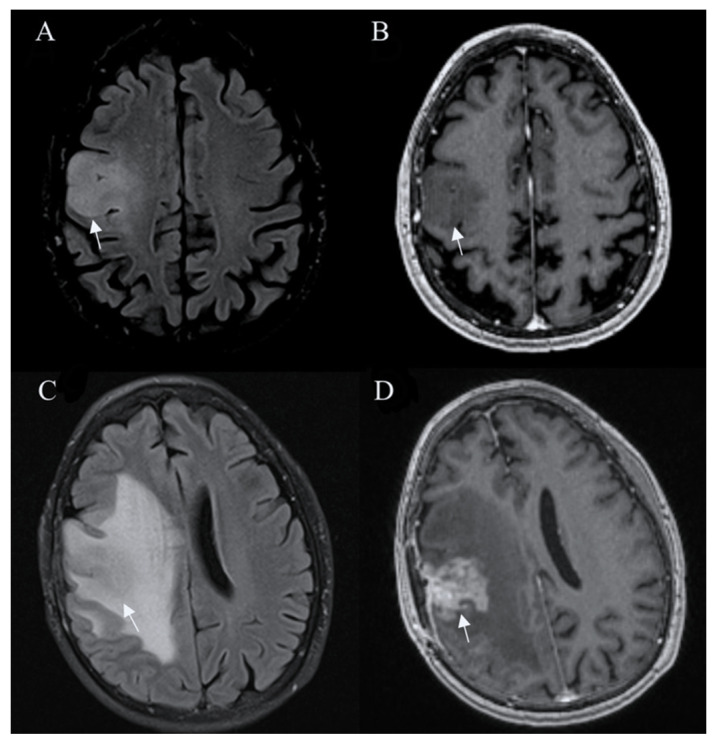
Glioblastoma IDH wild-type. Axial T2-FLAIR (A) and postcontrast axial (B) T1W images are suggestive of a low-grade tumor. However, followup FLAIR (C) and postcontrast T1W (D) images 6 months later show tumor progression with irregular contrast enhancement, surrounded by extensive edema, characteristic of glioblastoma. Arrows in A-D images shows the mass-like site of IDH wild-type glioblastoma. Images used for publication are taken from LUHS Radiology Clinic archives servers.
